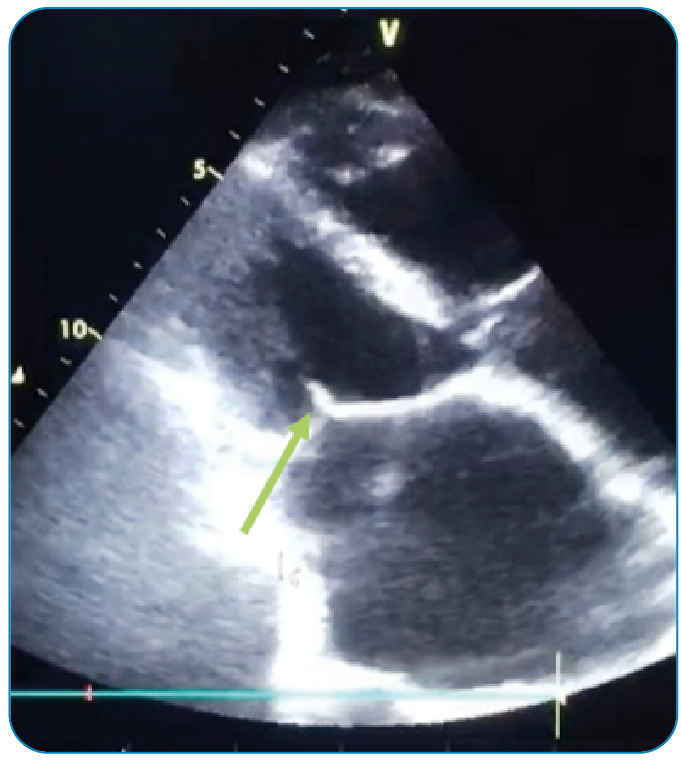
A transthoracic echocardiography image showing a vegetation on the anterior mitral valve.
